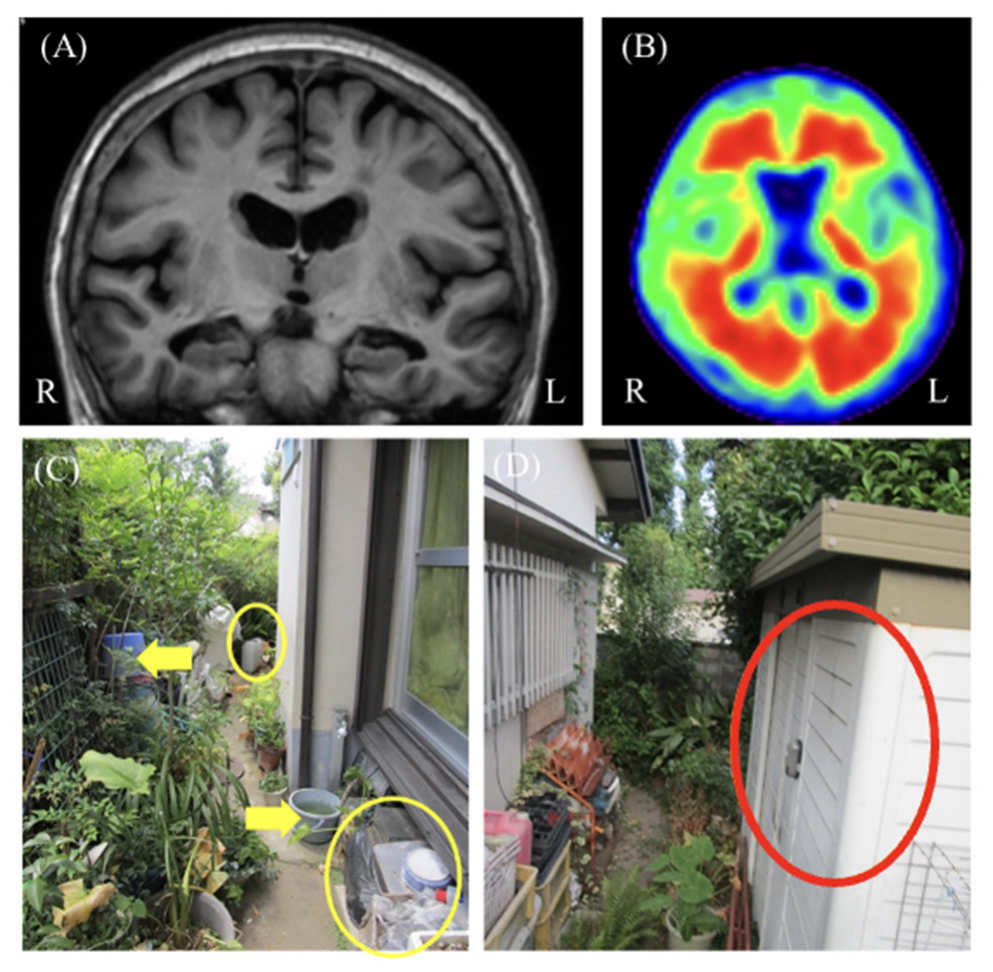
Case 2 images. (A) Brain magnetic resonance T1-weighted image showed bilateral hippocampal atrophy. (B) Florbetapir positron emission tomogrpahy showing positive results. (C,D) These images show the patient's cluttered garden. The yellow circled items were the examples of things she has not been used in a while. The yellow arrowed ones were the examples of duplicated items. We proposed to her family that they reduced these items after consulting with her (C). The red circled storage was one of the several storage places of her garden items. We proposed to her and the family that they limitedly stored the garden items in just this place (D).
